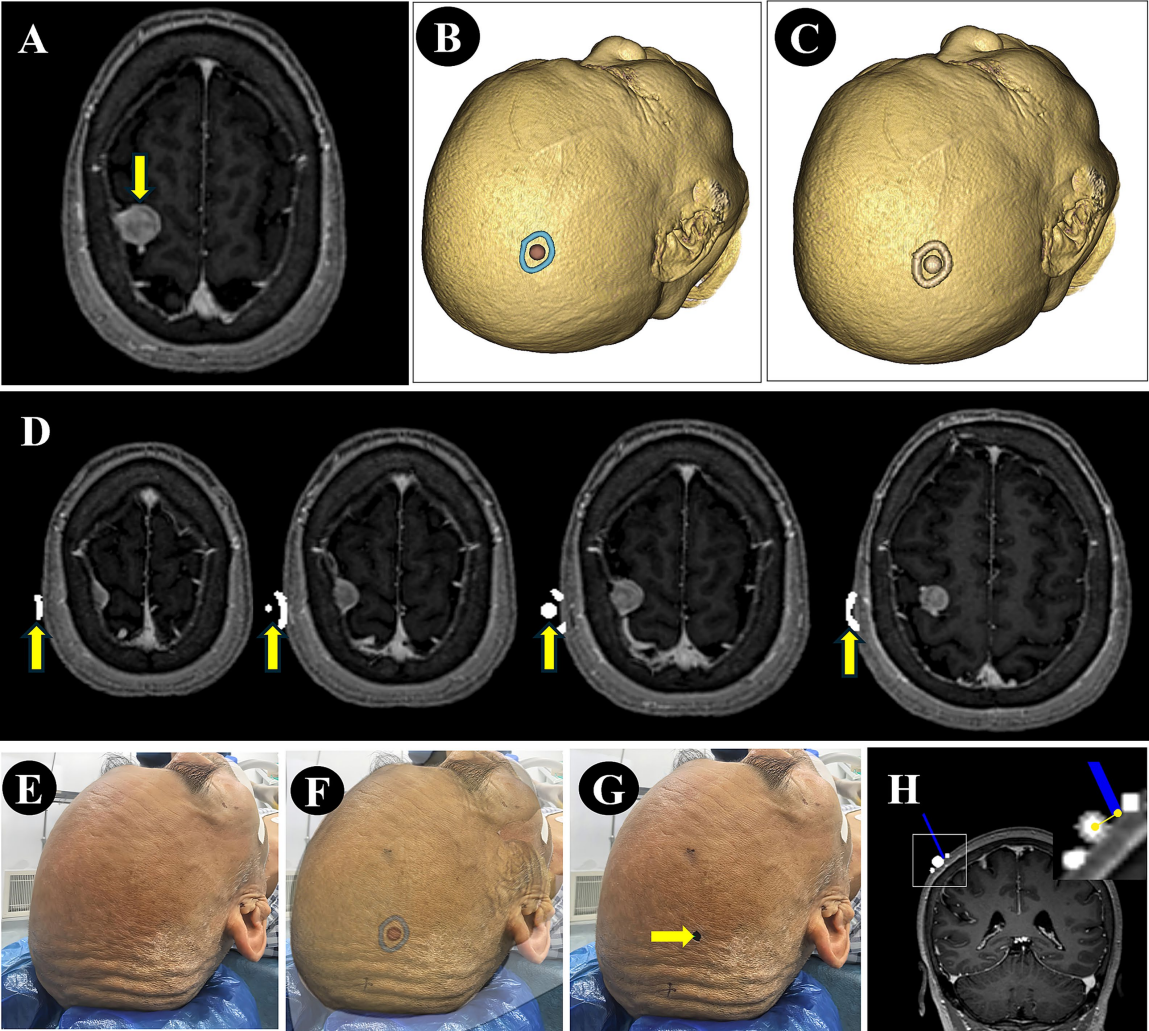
Image processing and error measurement. (A) A right parietal lesion was shown on preoperative MRI (purple arrow). (B) The lesion boundary (blue) and center point (red) were reconstructed from preoperative imaging and the view was saved for NP-Guide localization. (C) The lesion boundary and center point were filled in grayscale and fused with the original image. (D) The fused image was resampled and exported in DICOM format, containing the lesion boundary and center point (yellow arrow). (E–G) The patient’s head was fixed (E), the NP-Guide projection was applied (F), and the lesion center was marked on the scalp (green arrow, G). (H) An optical navigation probe (blue) was placed on the marked point, and the ONS was used to measure the distance between the probe tip and the true lesion center.
